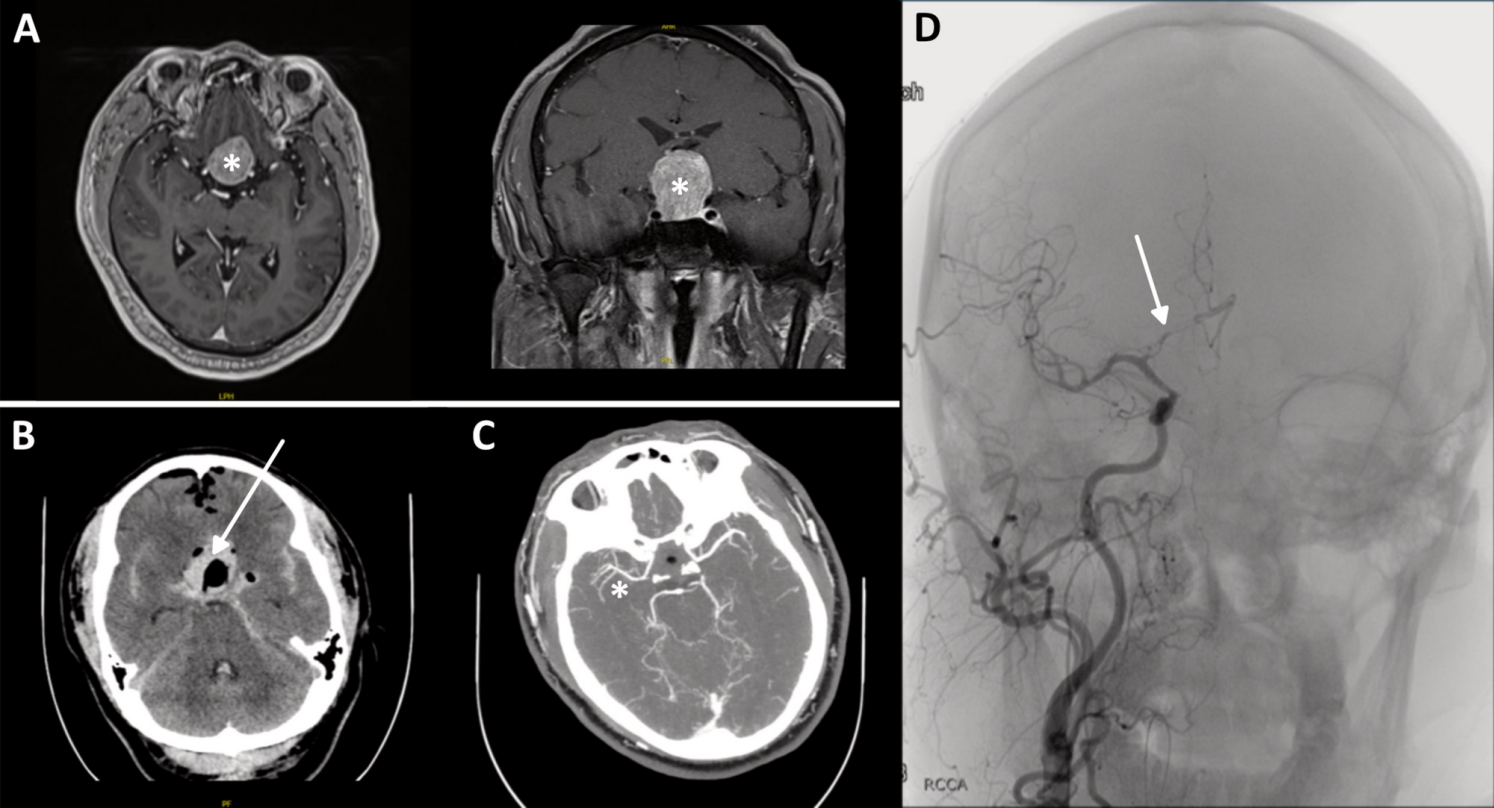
Case 2: 55-year-old man (A) Pituitary adenoma on preoperative MRI (asterisks). (B) CT of the head performed on POD 1 reveals SAH in the basal cisterns (arrow). (C) On POD 12, CTA of the head confirms vasospasm in the right MCA (asterisk). (D) Cerebral angiography performed on POD 14 reveals severe right ACA vasospasm (arrow), which was subsequently treated with intra-arterial nicardipine POD, postoperative day; CTA, CT angiography; MCA, middle cerebral artery; ACA, anterior cerebral artery
